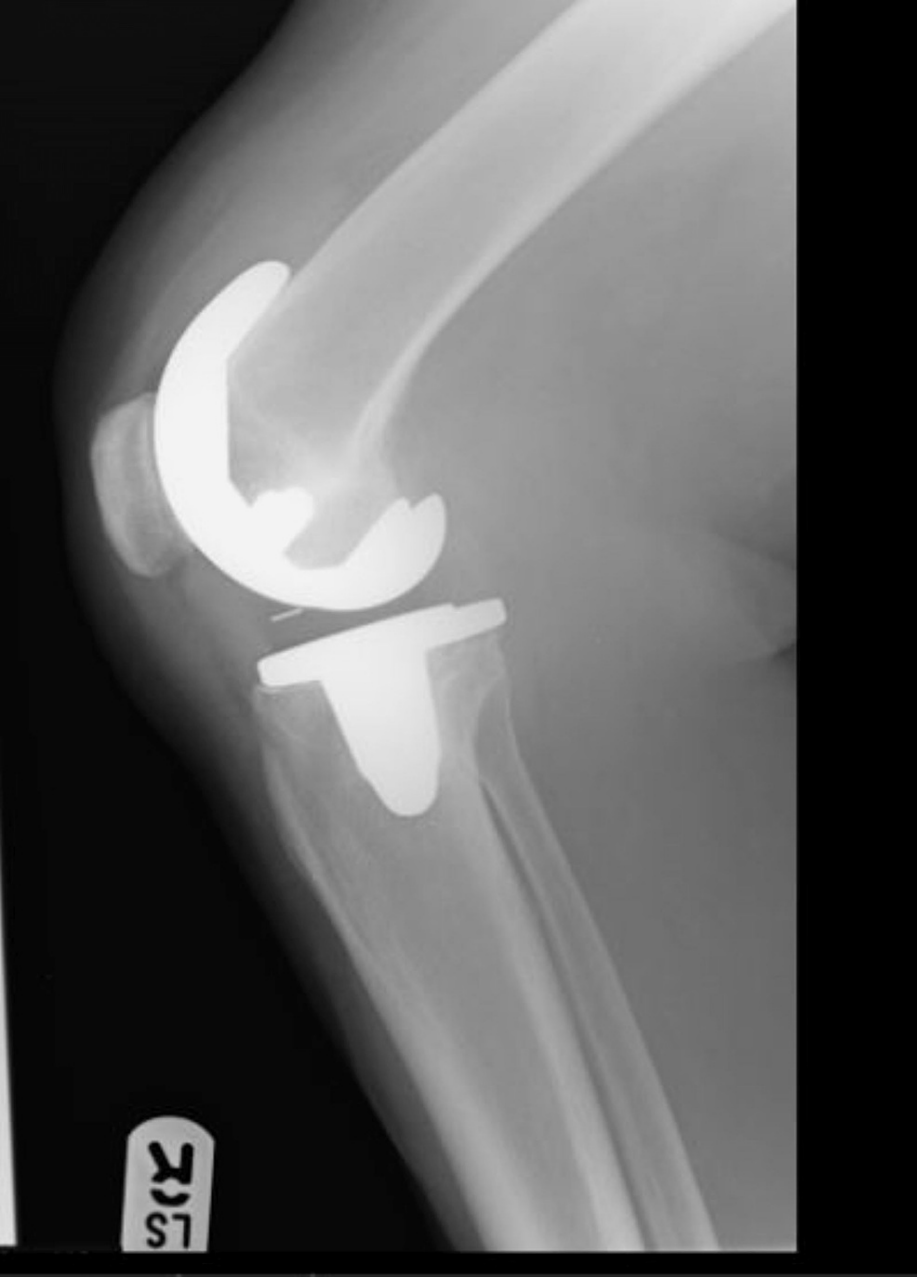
One year postoperative X-ray, lateral view
